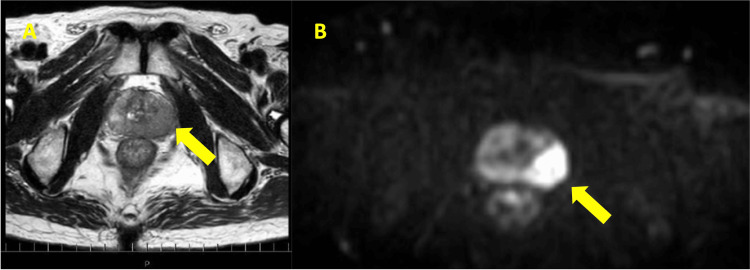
Pelvic MRI showing PI-RADS score 5 lesion A: Axial T2-weighted imaging (T2WI) of the pelvis demonstrates a hypointense signal lesion in the left lobe of the prostate (yellow arrow), suggestive of prostate cancer. The lesion measures approximately 20 mm and is primarily located in the peripheral zone of the left lobe. The extracapsular extension was suspected; however, there was no definitive evidence of seminal vesicle invasion. B: Diffusion-weighted imaging (DWI) reveals a large area of diffusion restriction in the peripheral zone of the left lobe (yellow arrow), further supporting the suspicion of malignancy. PI-RADS: Prostate Imaging Reporting and Data System
